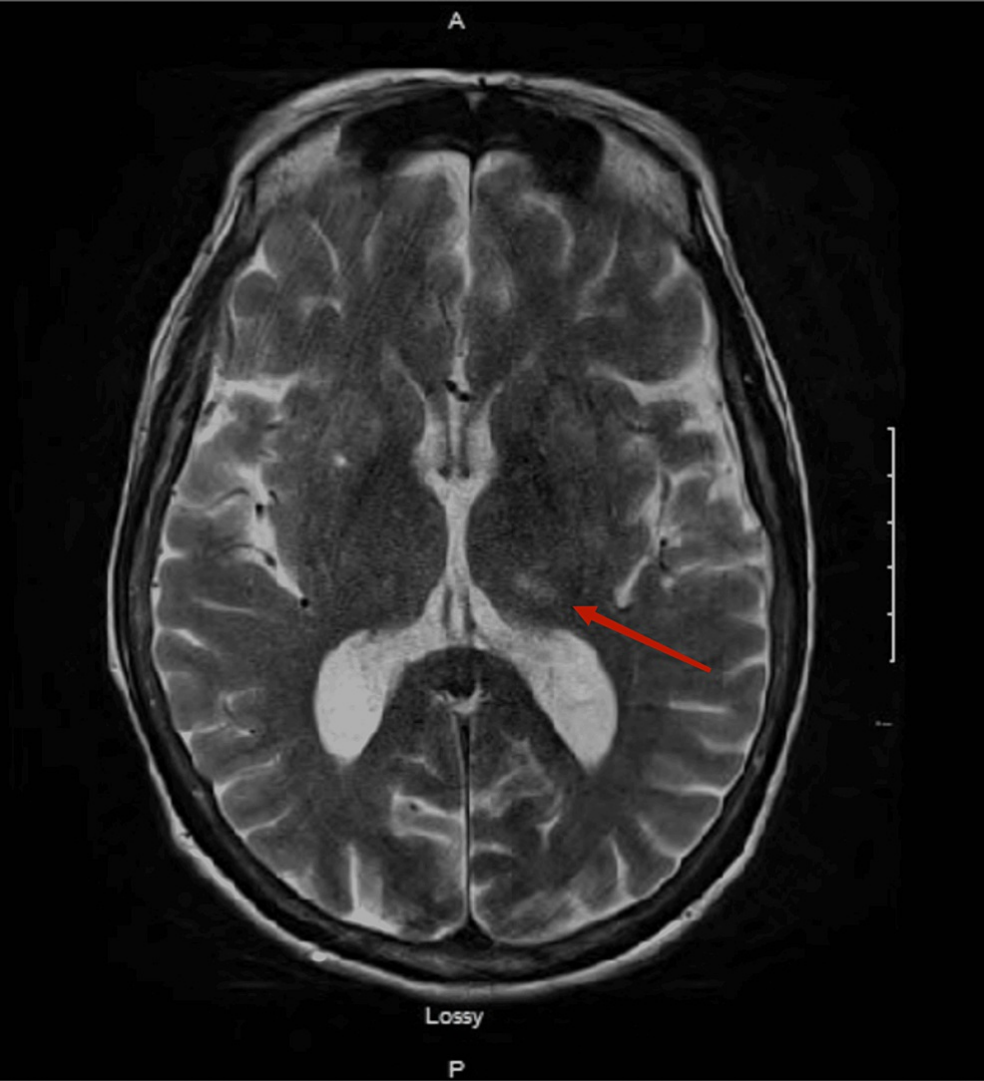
MRI brain and brainstem without contrast Increased T2 signal within bilateral thalami (left > right)
